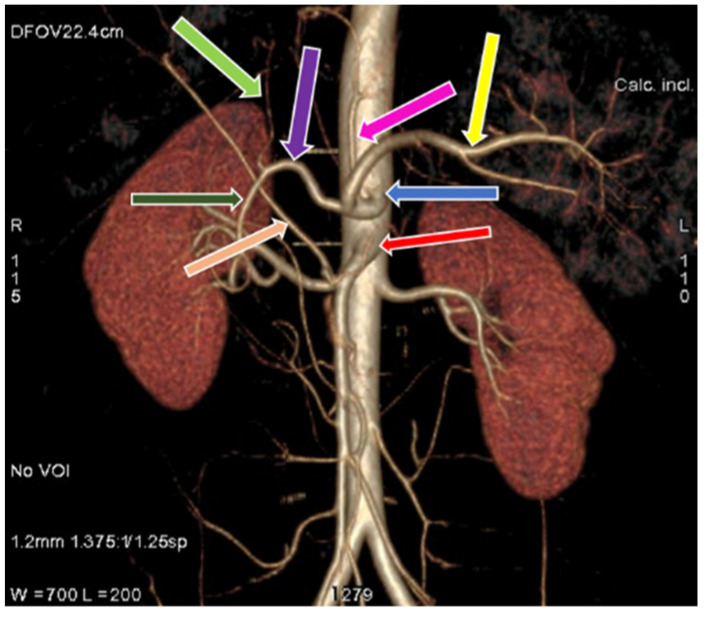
3D-VR image of (64 row scanner CT angiography) abdominal region showing celiac trunk (blue arrow) and superior mesenteric artery (red arrow), common hepatic artery divides into gastroduodenal artery (dark green arrow), left hepatic artery (light green arrow). Replaced right hepatic artery (light pink arrow) originated from superior mesenteric artery. Splenic artery (yellow arrow), left gastric artery (pink arrow), common hepatic artery (purple arrow).
